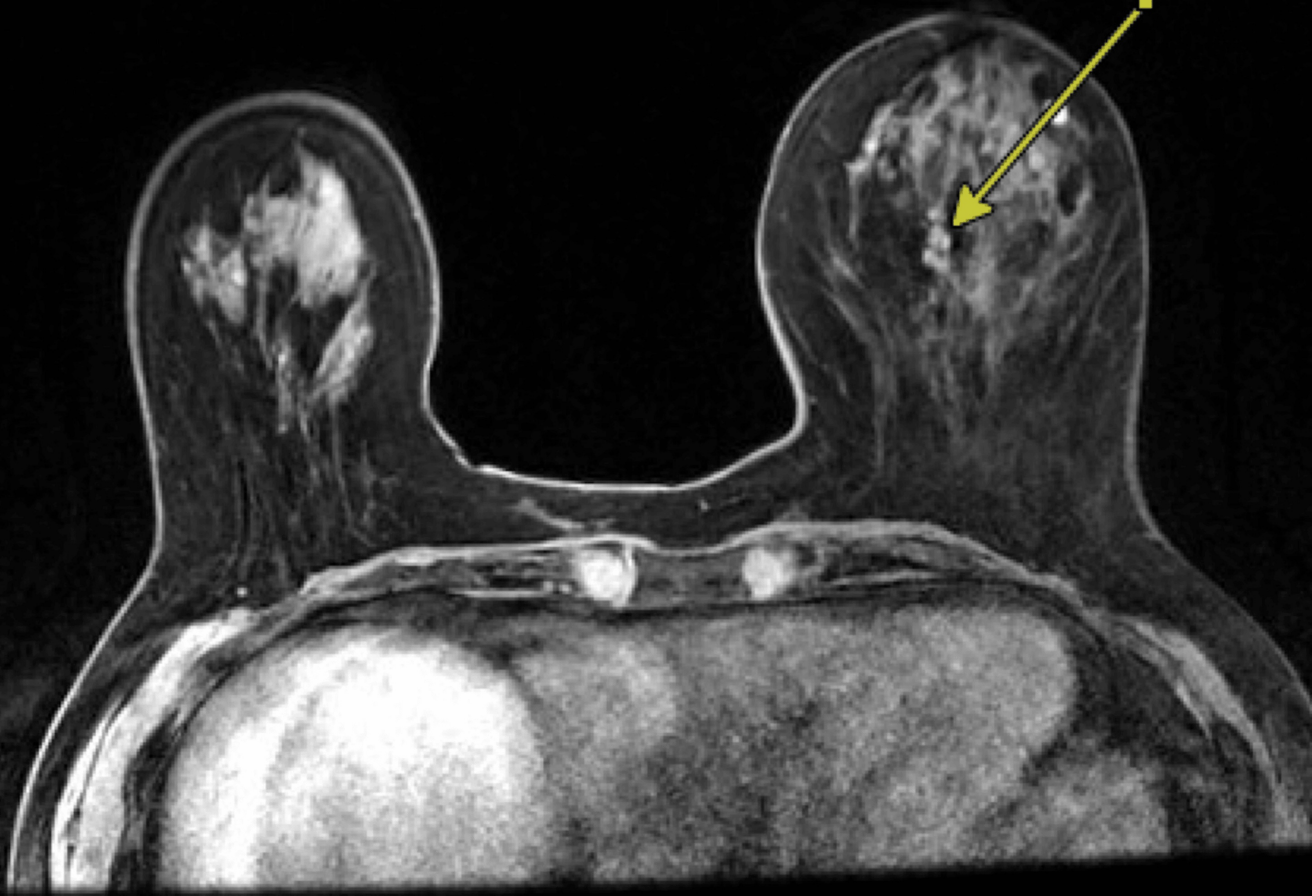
MRI redemonstrating a left-sided breast mass Arrow pointing to the mass for biopsy MRI, Magnetic Resonance Imaging
